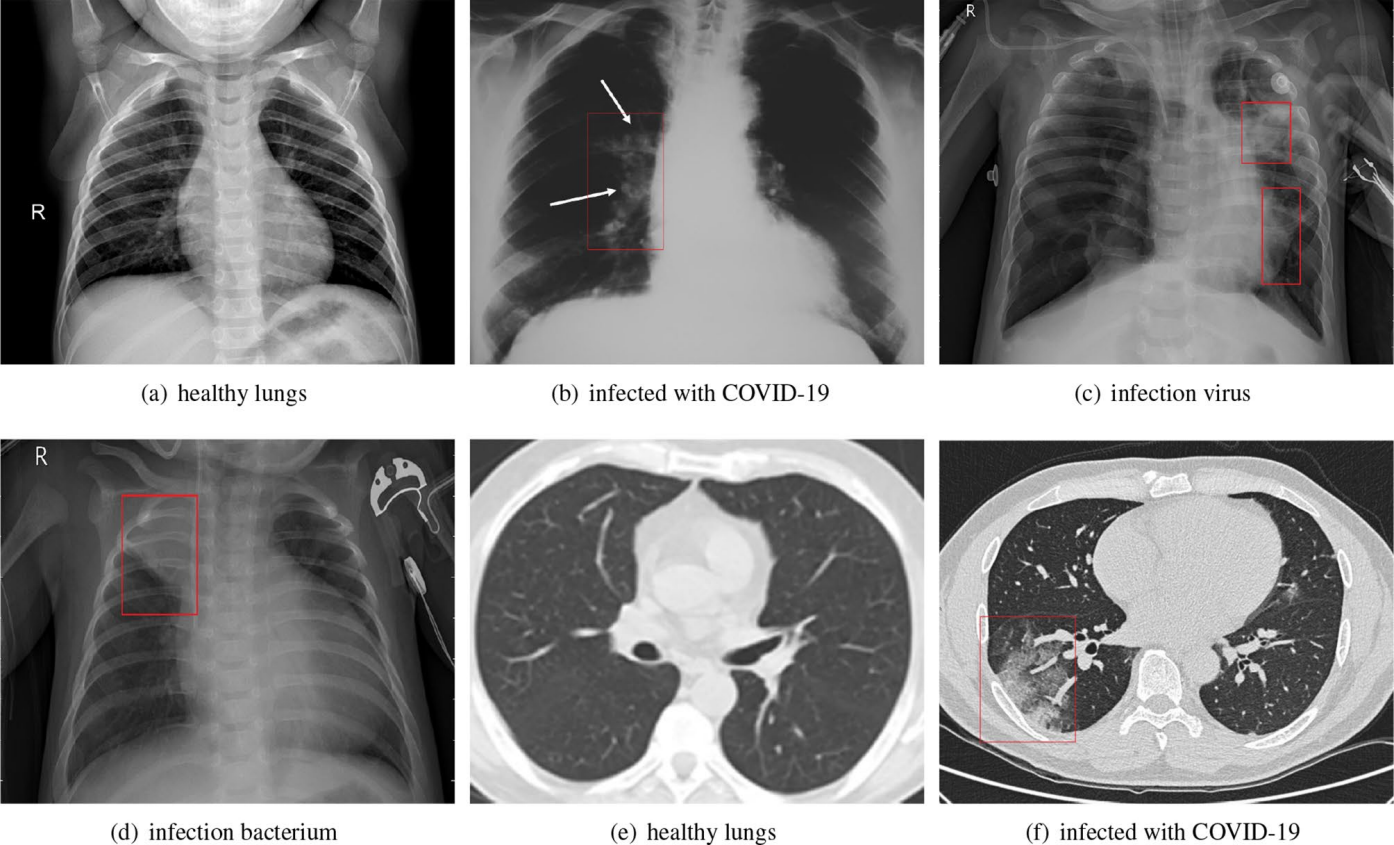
X-ray images (a–d) and CT images (e–f).
